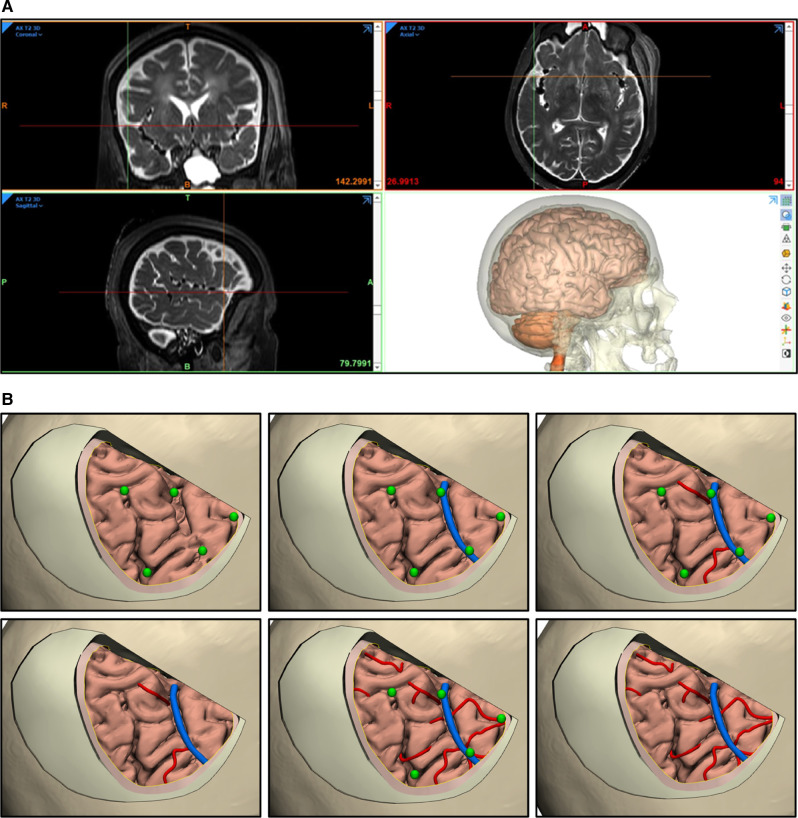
Adding vasculature to 3D virtual models. (A) Vascular structures are visible on MRI (coronal, top left; axial, top right; sagittal, bottom left). However, the delineation of vascular structures in 3D models (bottom right) is not practical or accurate unless a contrast medium is used before the vessels are injected. (B) An alternative method is to artificially add or draw arteries (*red lines*) or veins (*blue lines*) using certain anatomical landmarks acquired via neuronavigation and photography (*green dots*). *Used with permission from Barrow Neurological Institute, Phoenix, Arizona*.
